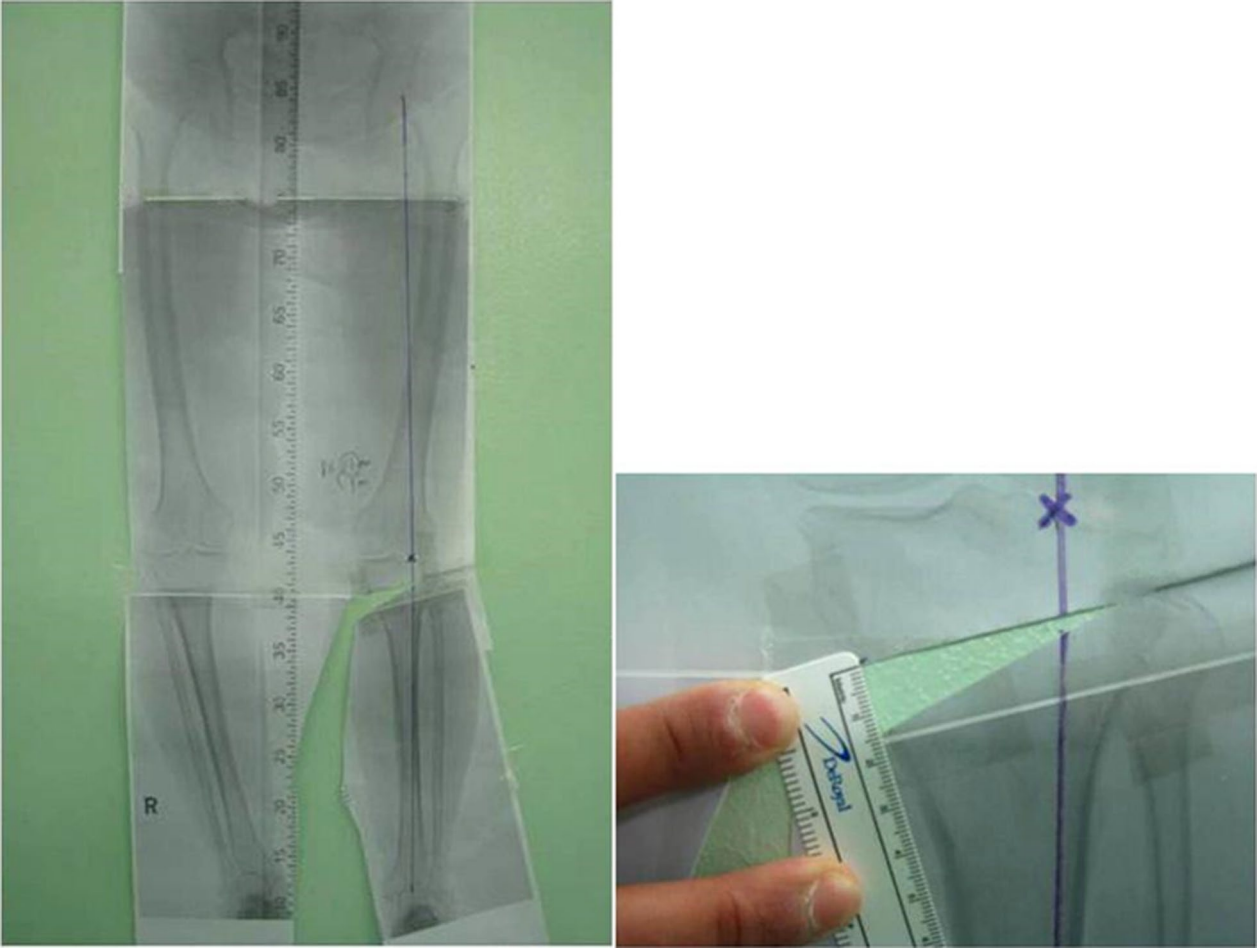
Real-size weight-bearing scanogram method. With a full-size radiograph printed on paper, a line is cut along where the osteotomy is going to be performed. The tibia is rotated until the weight-bearing line passed through the target point. Reprinted with permission from Lee DH: The weight-bearing scanogram technique provides better coronal limb alignment than does the navigation technique in open high tibial osteotomy. The Knee. Copyright © 2012 Elsevier B.V. All rights reserved
